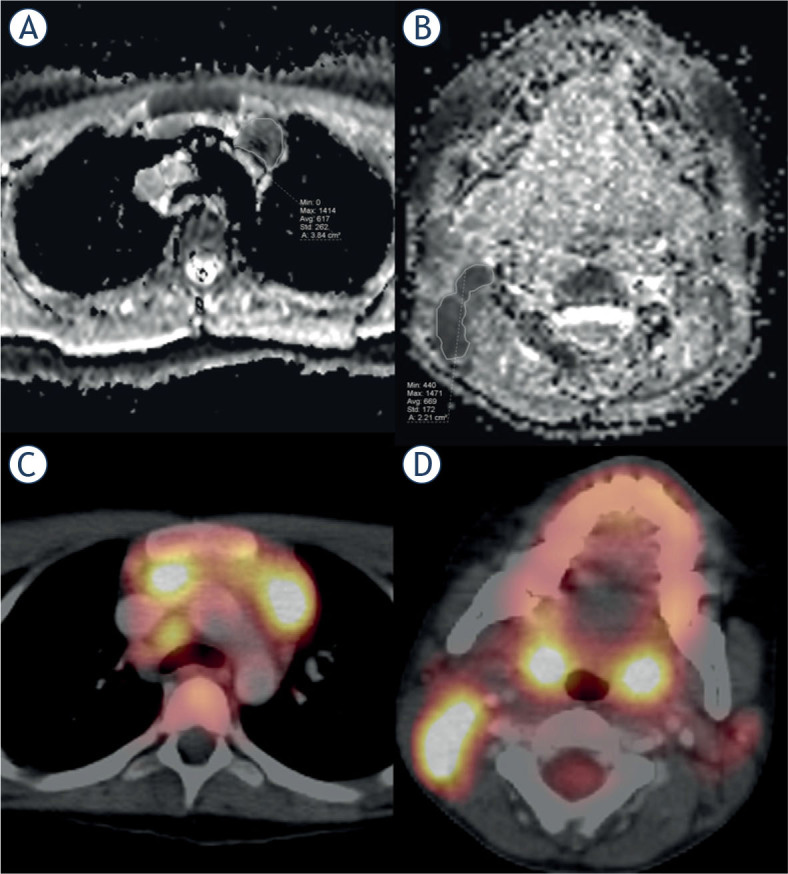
MRI and FDG-PET/CT imaging of two Hodgkin lymphoma (HL) patients. Left side (A, B): 12yo patient with newly diagnosed HL, (A) MRI examination with thoracal apparent diffusion coefficient (ADC) map. (B) correlating FDG-PET/CT examination with a HL lesion and calculated SUVmax. right side (C, D): 9yo patient with newly diagnosed HL, (C) MRI examination with neck ADC map. (D) correlating FDG-PET/CT examination with a HL lesion and calculated SUVmax.
